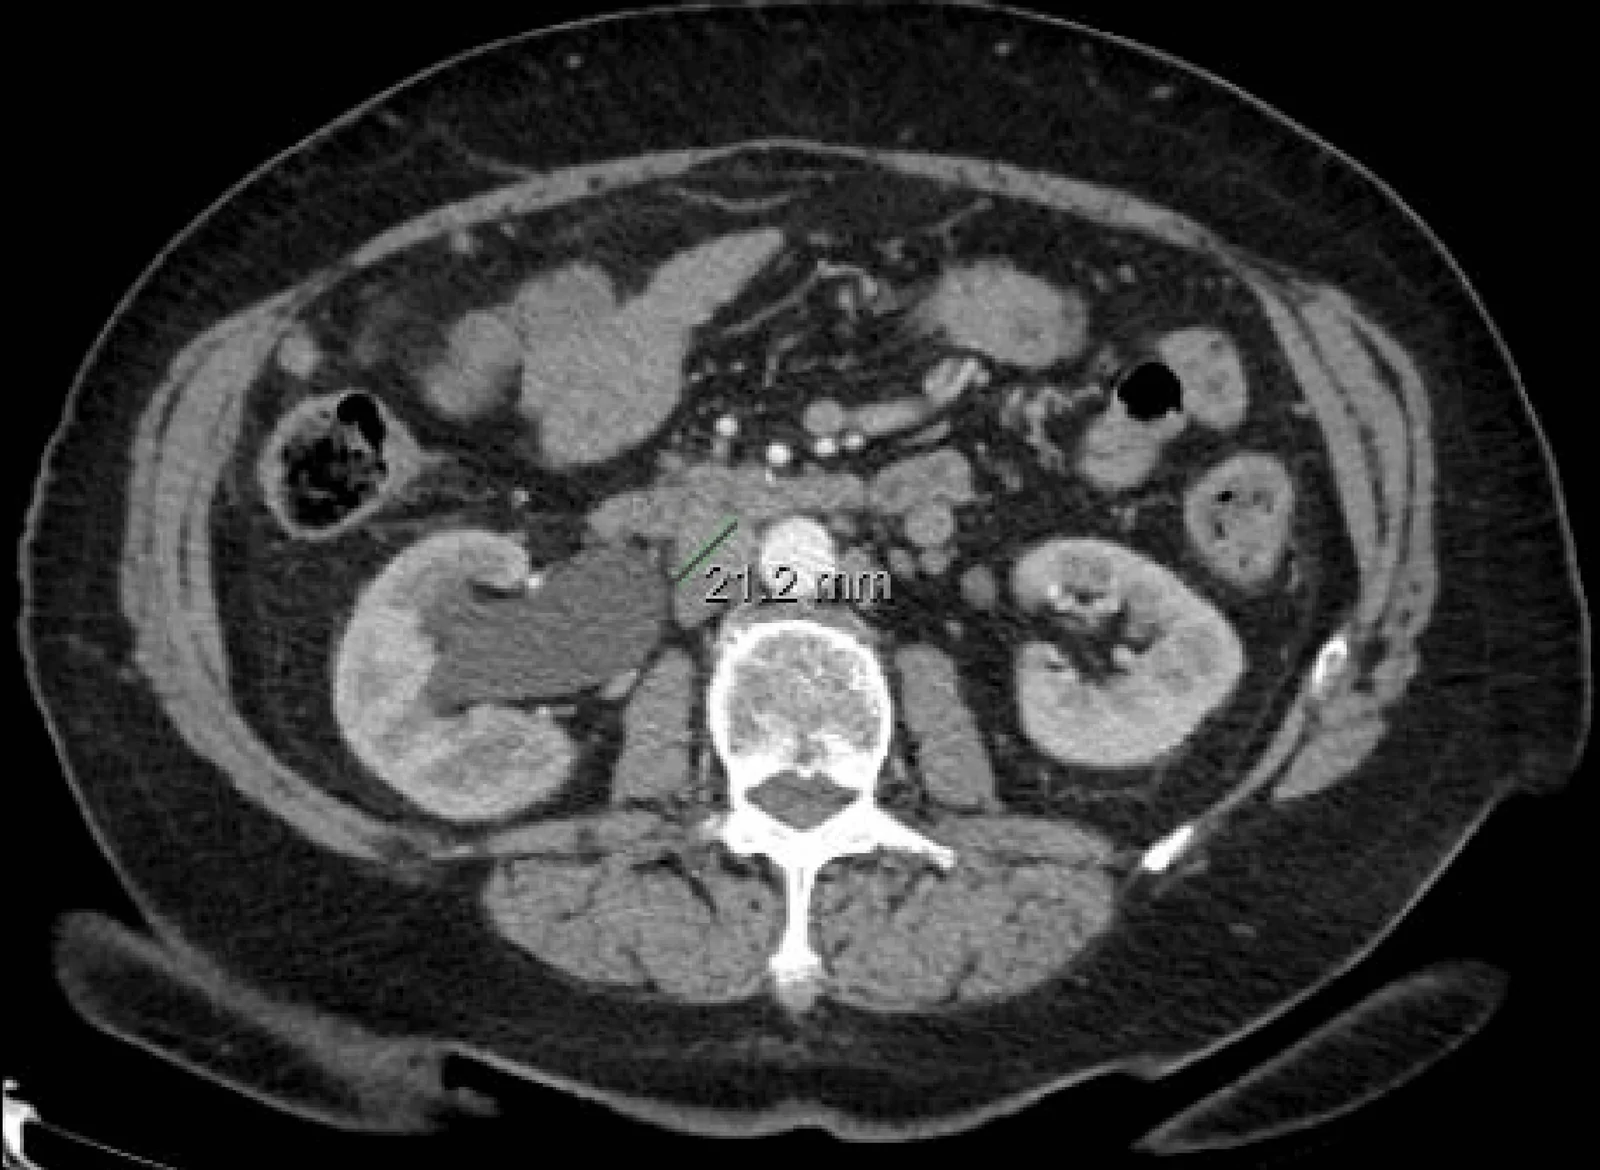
Restaging CT after with one cycle of maintenance 5fu-lucovorin demonstrated increased retroperitoneal lymph nodes, measured at 21.2 mm
